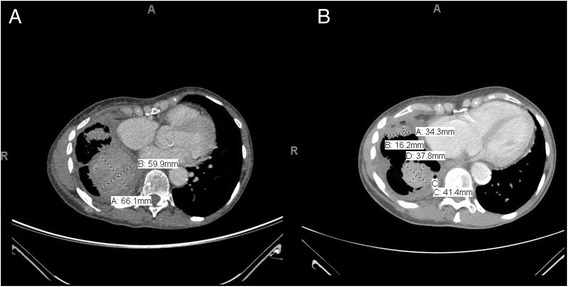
Chest CT Scans of Patient on Ceritinib Tratment. a Chest CT Scan Prior to Ceritinib Treatment. b Chest/Upper Abdomen CT Scan Post Ceritinib Treatment
